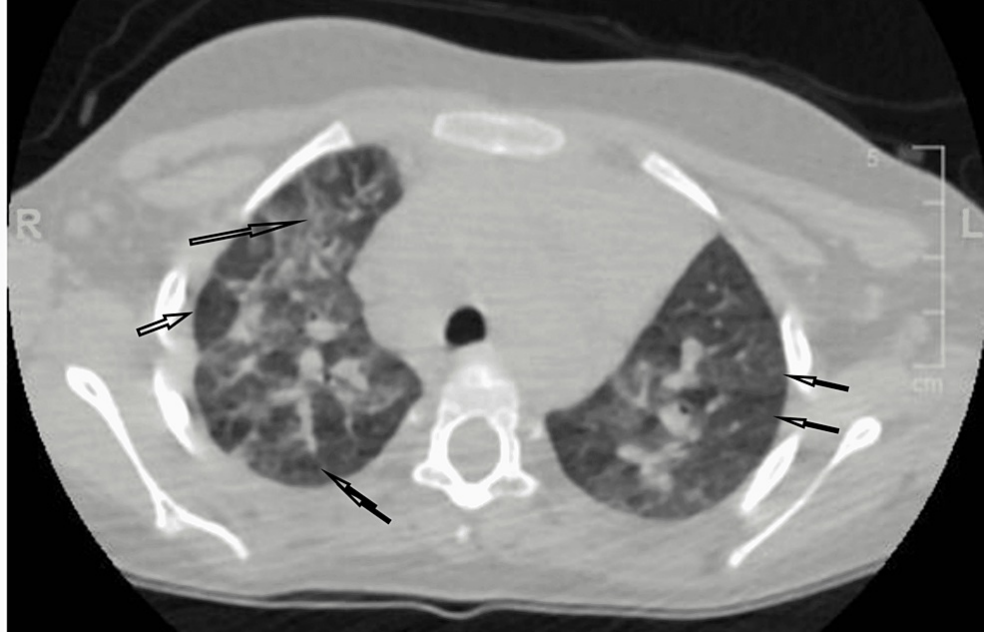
CT chest showed bilateral multiple patchy areas of differential pulmonary attenuation (mosaic pattern of lung attenuation) (black arrows).
